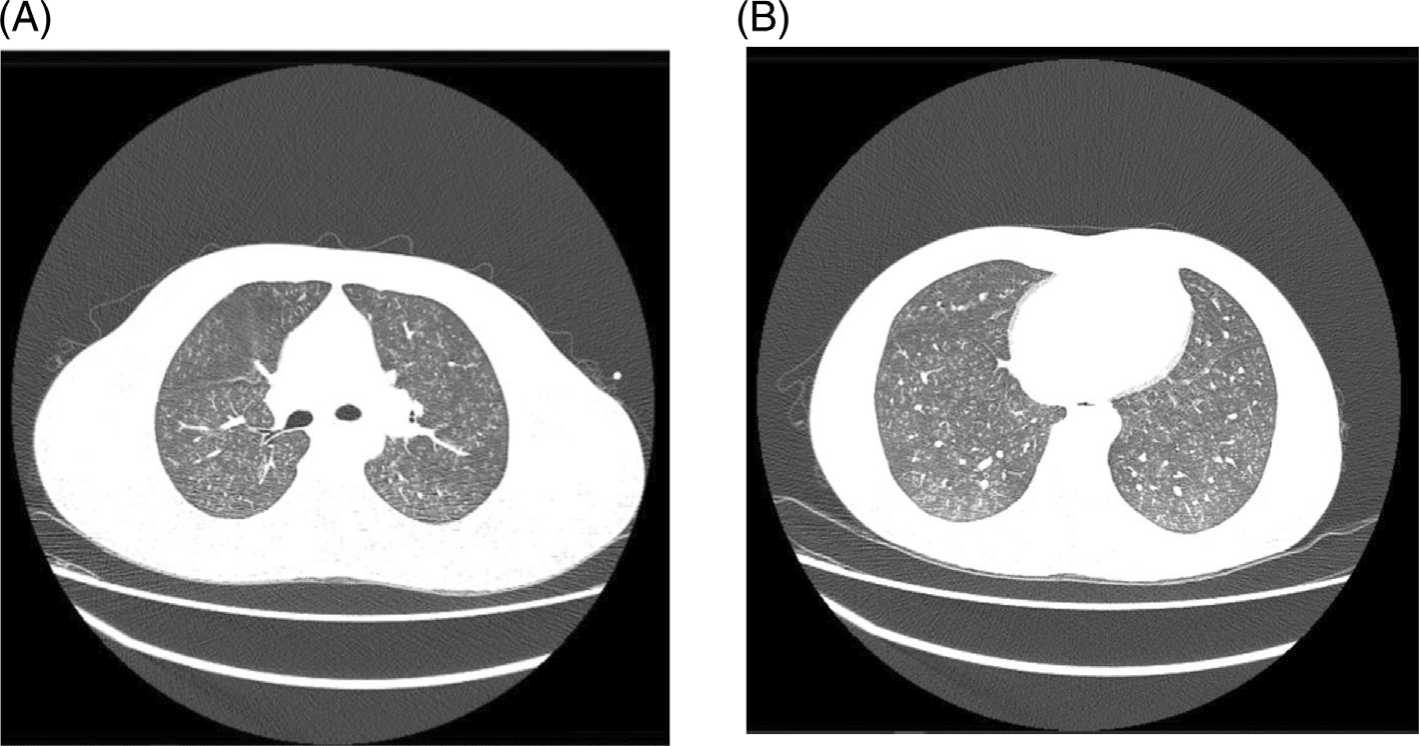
(A, B) High‐resolution computed tomography thorax (axial view) showed diffuse ground‐glass centrilobular nodules with minimal peripheral bronchiole wall thickening.
